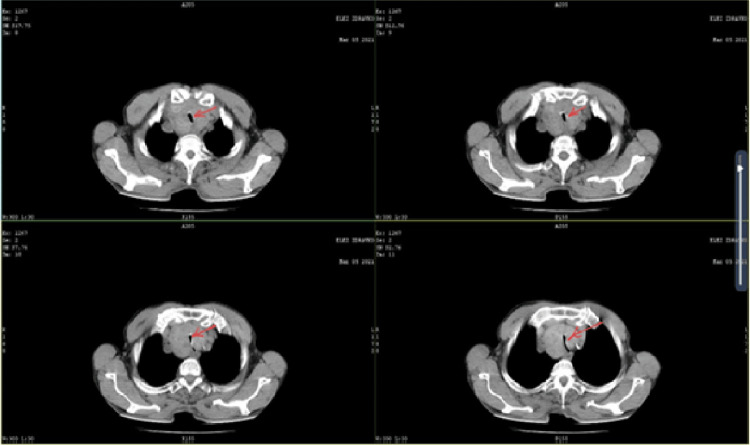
Computed tomography (CT) of the neck and chest, taken four days before surgical treatment, showing tracheal compression and airway obstruction caused by the surrounding thyroid gland tissue.
